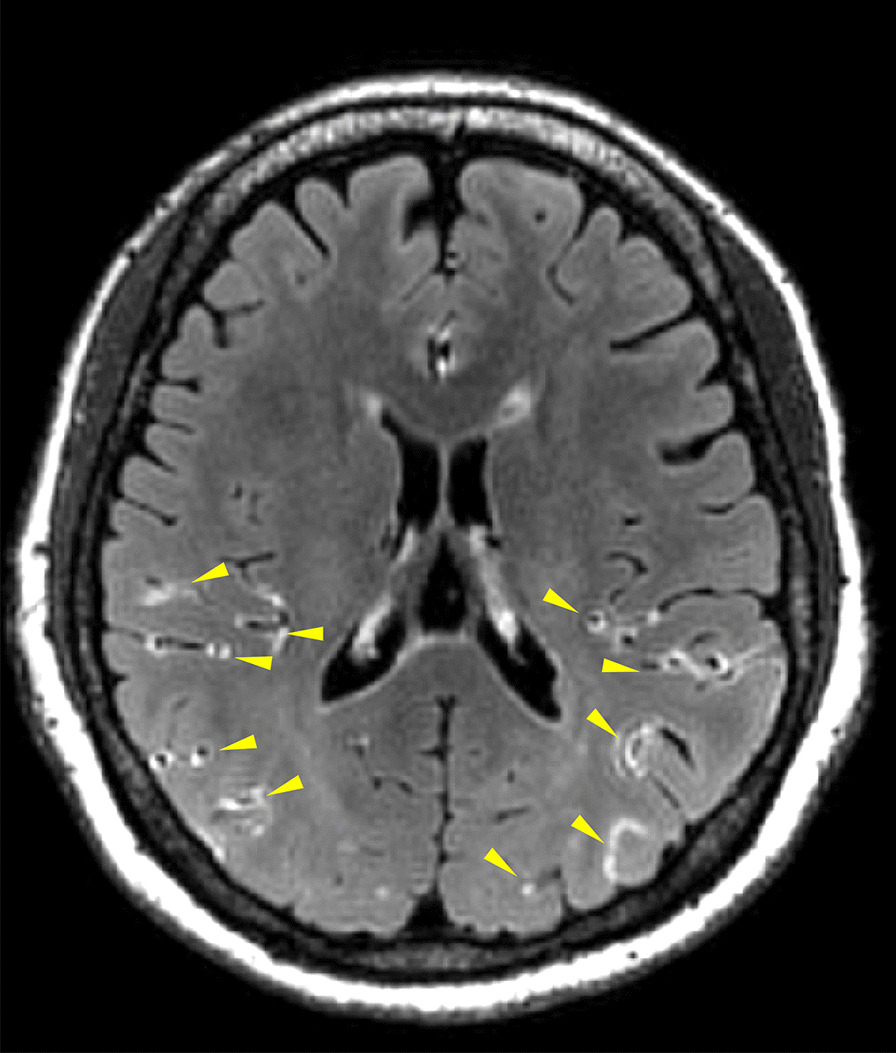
Typical imaging finding of blood–brain barrier breakdown demonstrated by contrast-enhanced FLAIR imaging. The gadolinium-based contrast medium extravasated from the cerebral vessels to the cortical sulci appears hyperintense on FLAIR imaging (yellow arrow heads), providing macroscopic imaging evidence of BBB breakdown
